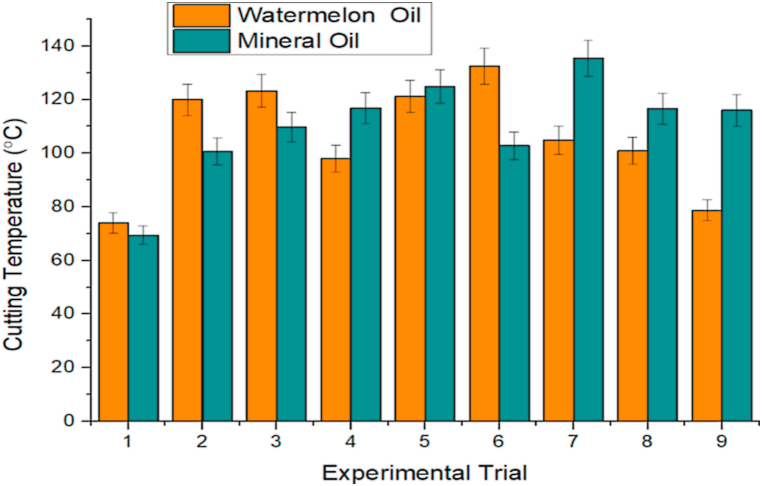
Effect of machining lubricants on CT
